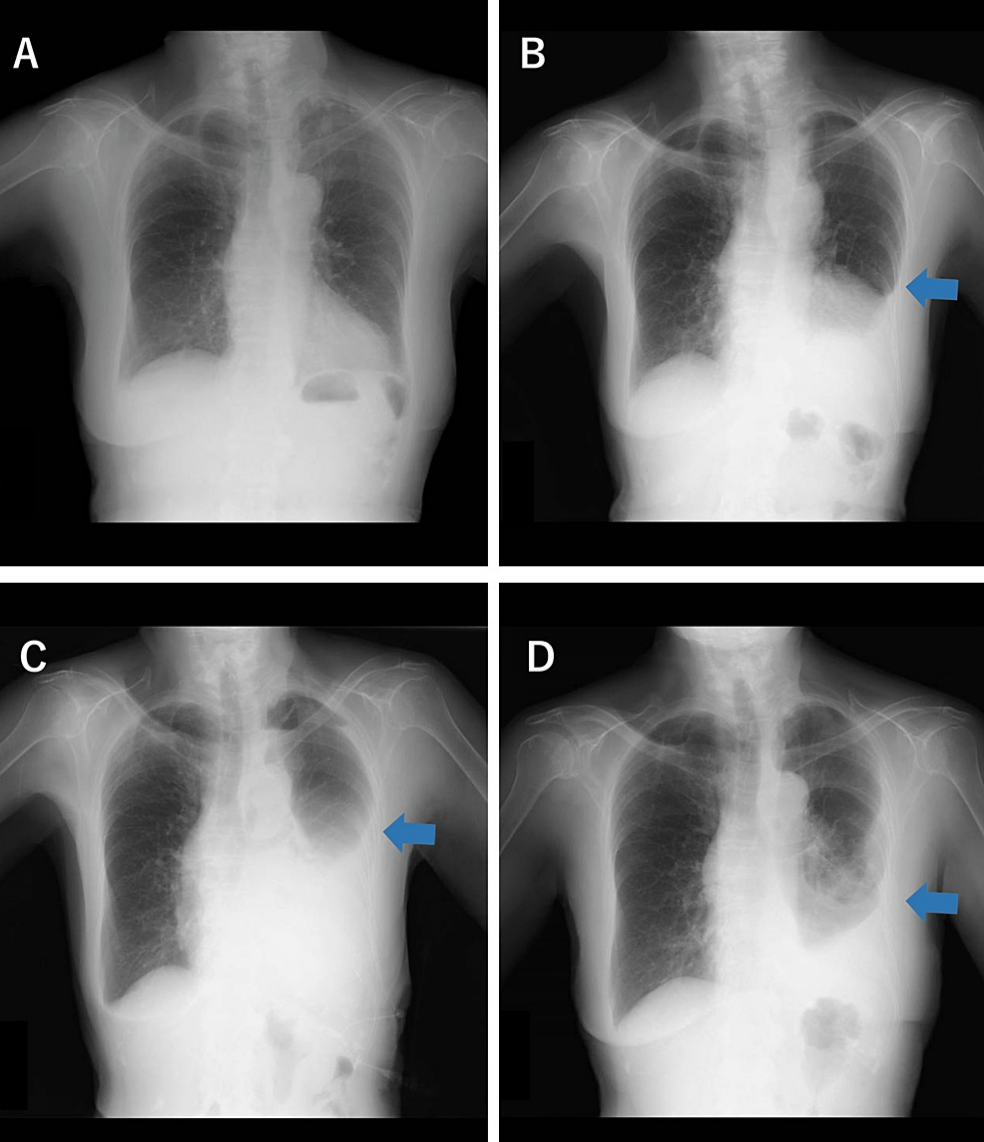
Chest X-ray Chest X-ray of the patient (A) before radiotherapy, (B) 18 months after radiotherapy, (C) 36 months after radiotherapy, and (D) 46 months after radiotherapy. Blue arrows indicate the pleural effusion.
